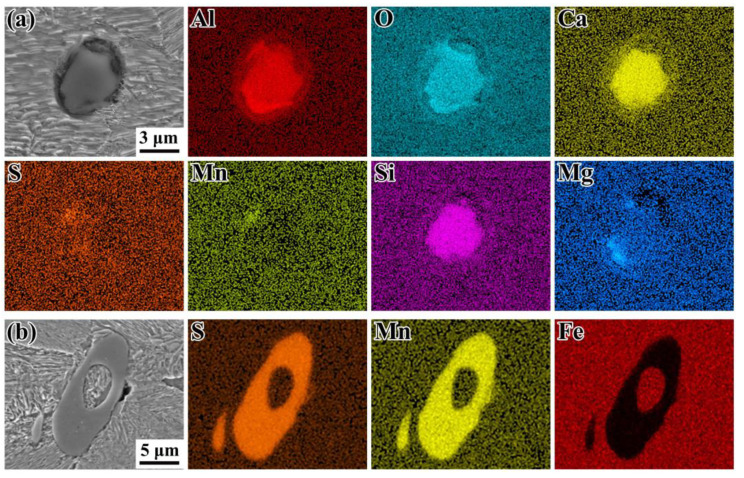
SEM images and EDX (energy dispersive X-ray analysis) maps of the oxysulfides: (a) Al_2_O_3_ surrounding oxide, (b) elongated MnS inclusion.
